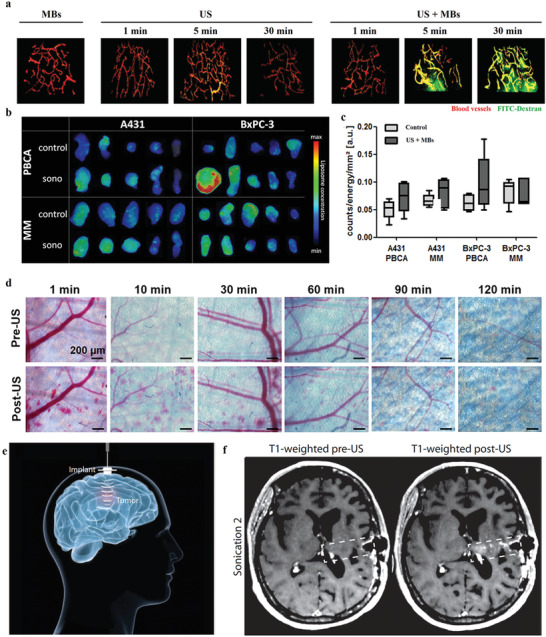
a) The effect of the vascular permeability for brain blood vessel with MBs treatment under ultrasonic activation upon 1 min, 5 and 30 min. Reproduced with permission.^[^
